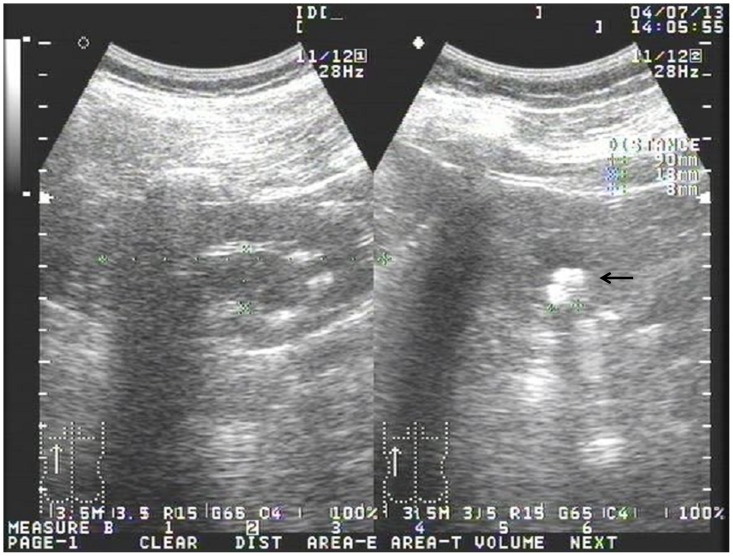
Sonography of the left kidney. A hyperechoic mass measuring 8(right panel, arrow).
